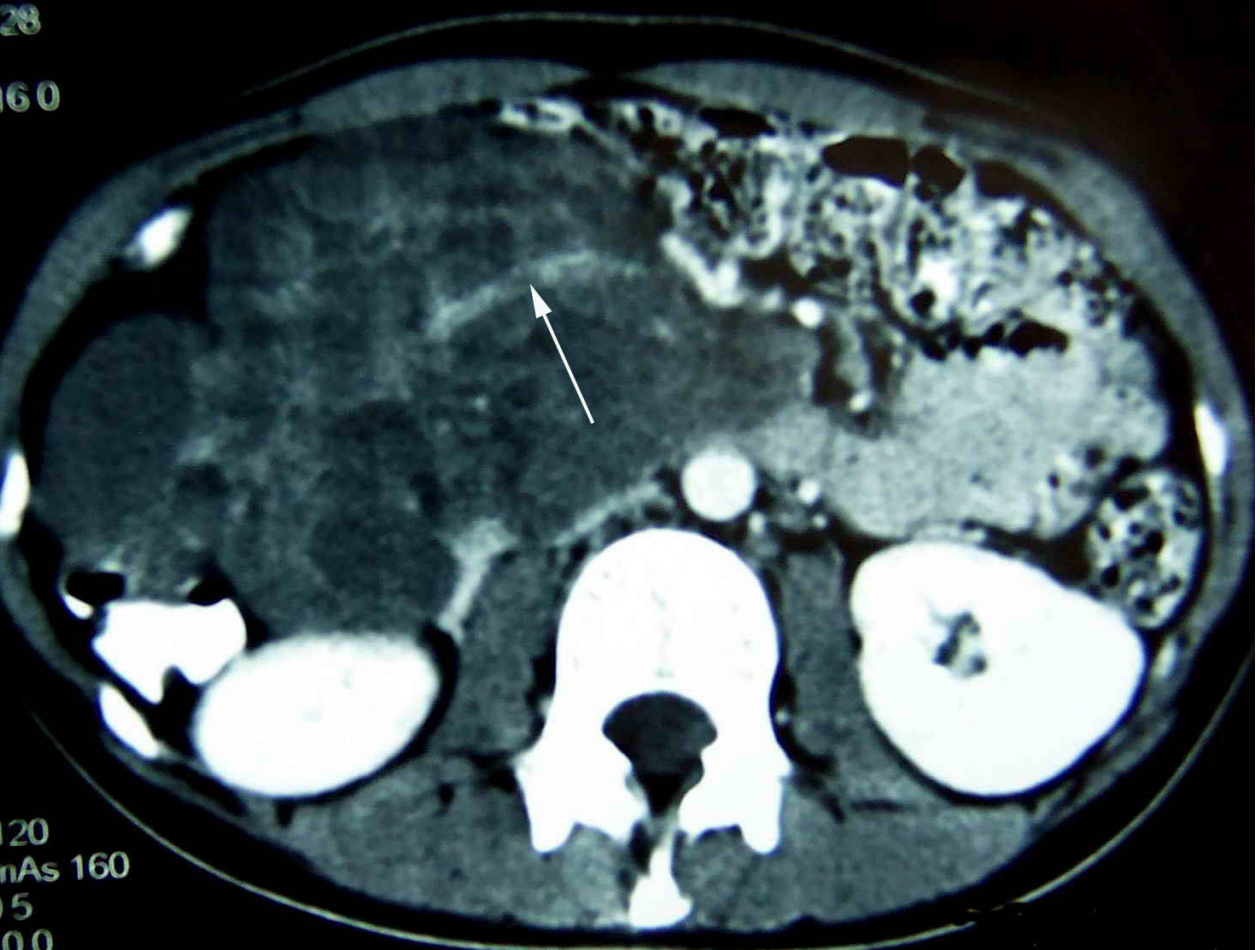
Abdominal Computed tomography demonstrating a large tumour with partial blood flow(arrow) in abdominal cavity.
